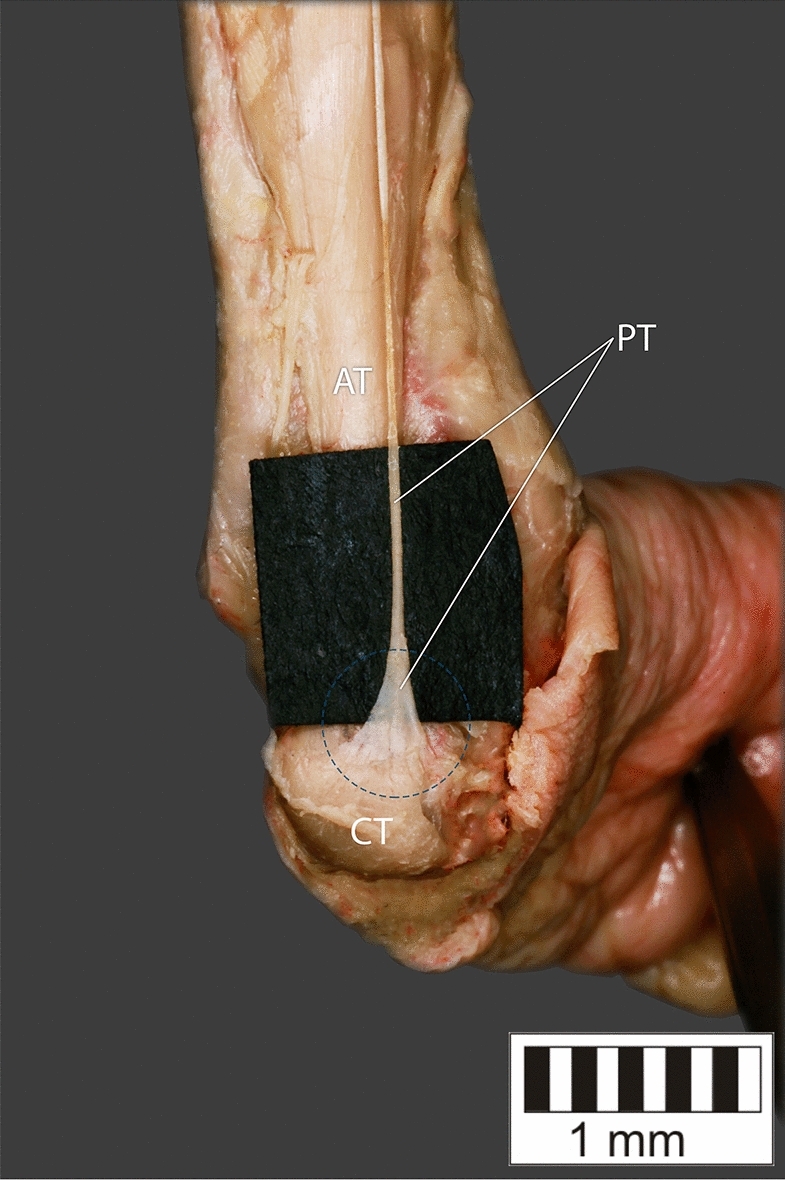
Type V of plantaris tendon. *CT*- calcaneal tuberostity, *AT* Achilles tendon, *PT* plantaris tendon.
